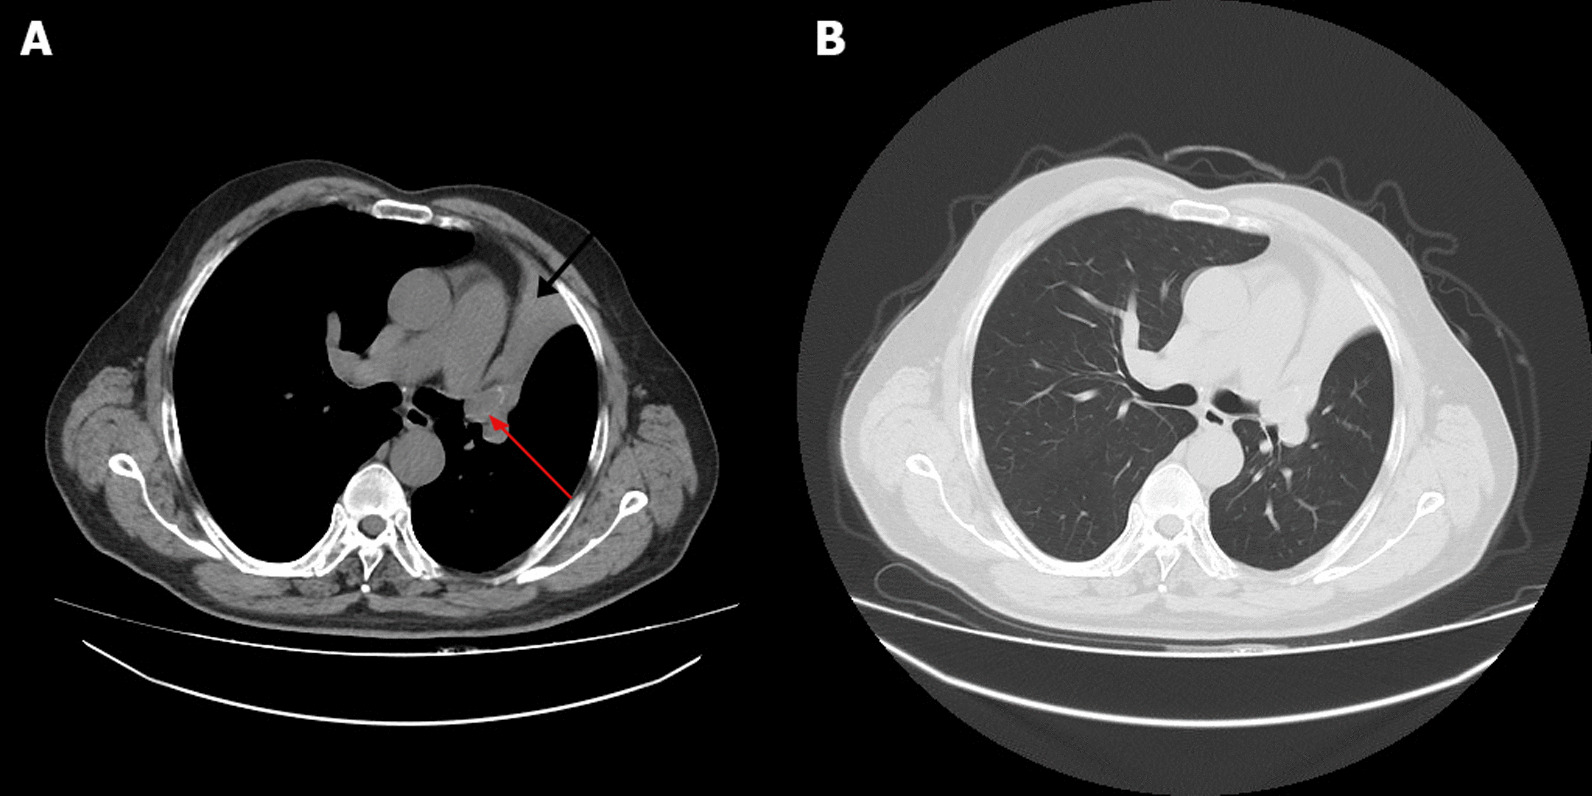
A Axial chest CT scan revealing soft-tissue mass measuring approximately (23 × 17) mm within the left main bronchus (red arrow) and atelectasis of the anterior segment of the left upper lobe (black arrow). B CT scan with chest window showing no lung parenchyma involvement or abnormalities
